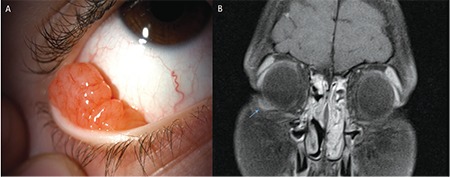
A) The salmon-colored lesion at the inferotemporal conjunctiva. B) The mass lesion on the right inferolateral region on T1 fat-suppressed MRI (arrow)
